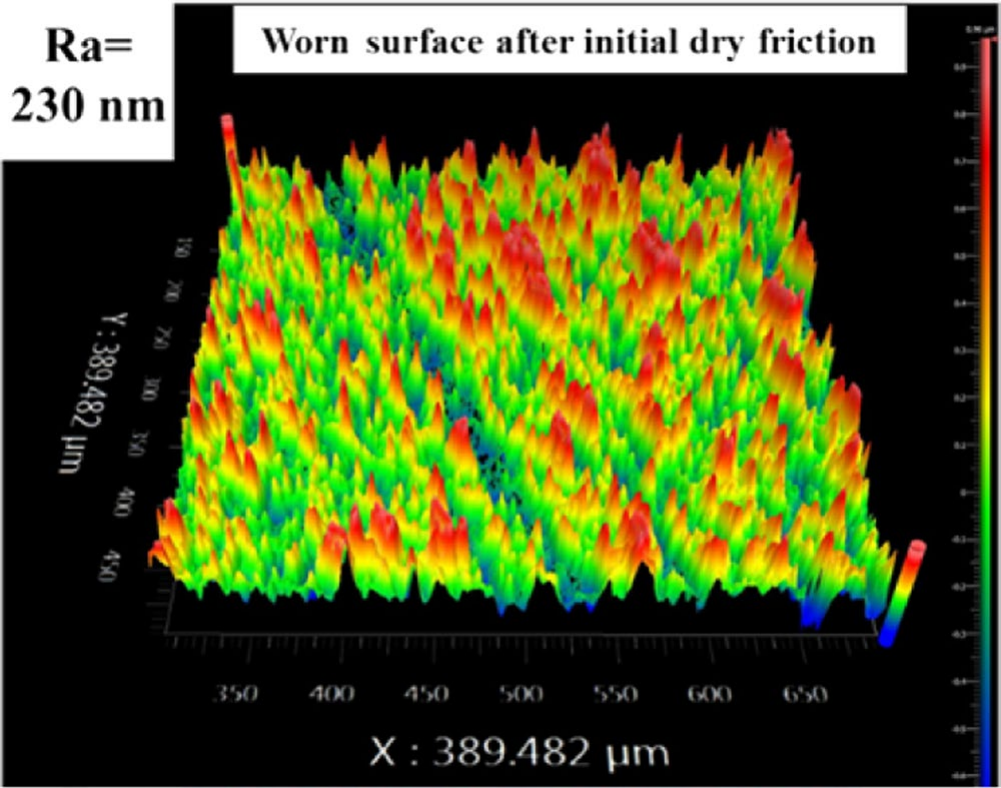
Worn surface topography after ultrasonic cleaning after initial dry friction: The surface is more coarse, and the peak of asperities obviously increases; the surface roughness is 230 nm.
